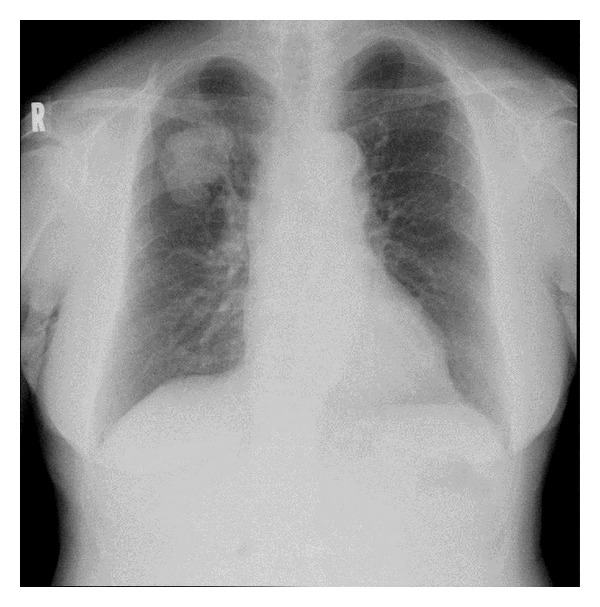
Routine chest X-ray examination shows a large coin lesion in the right upper lung field.
